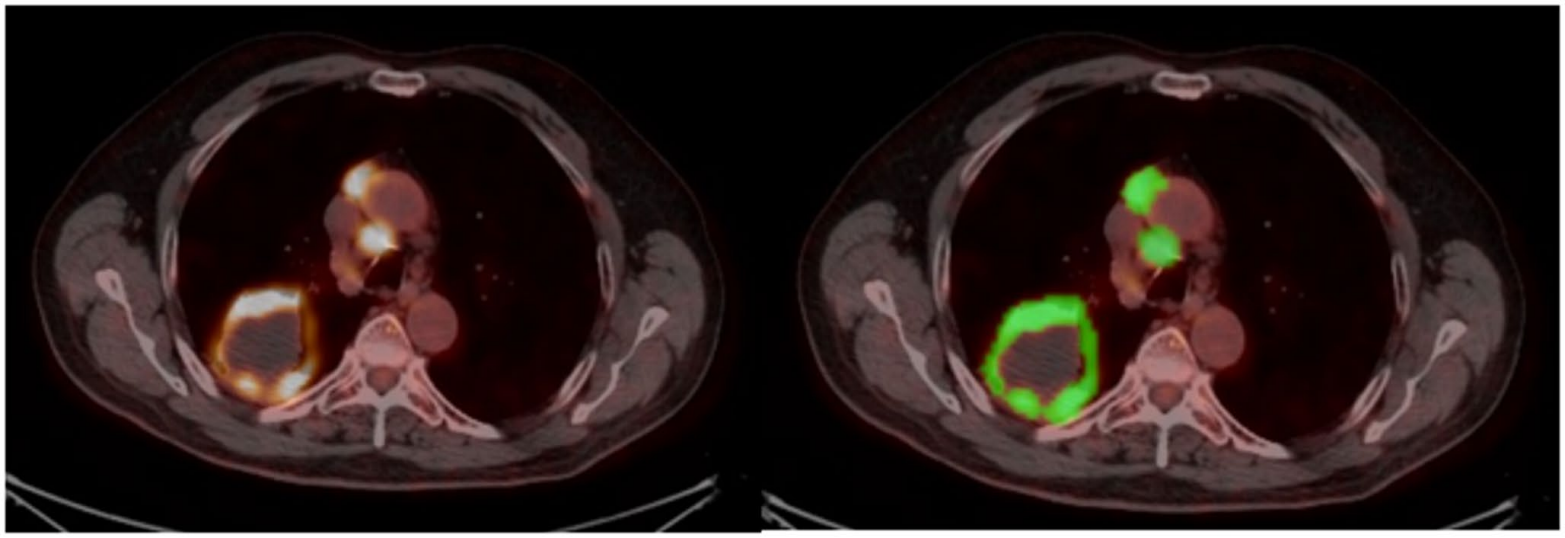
Representative examples of lesion segmentation and volumetric/dissemination metrics derived from pre-treatment 18 F-FDG PET/CT. *Left panel*: Fused PET/CT images of a patient showing a primary lung mass with mediastinal lymph node metastases. *Right panel*: Corresponding semi-automatic segmentation of metabolically active tumor lesions using a fixed SUV threshold of 2.5, with volumes of interest (VOIs) displayed in green
